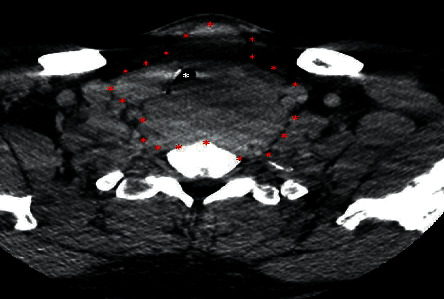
Enhanced axial computed tomography image at the level of the upper trachea demonstrating a large thyroid mass (red asterisks) resulting in compression and right lateral displacement of the trachea (white asterisk).
